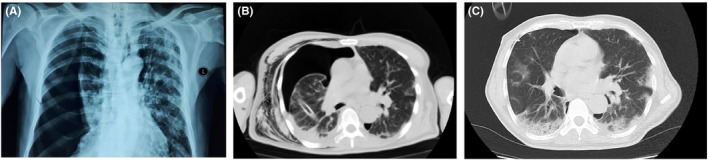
Chest CT scans and chest X‐ray of case 4
